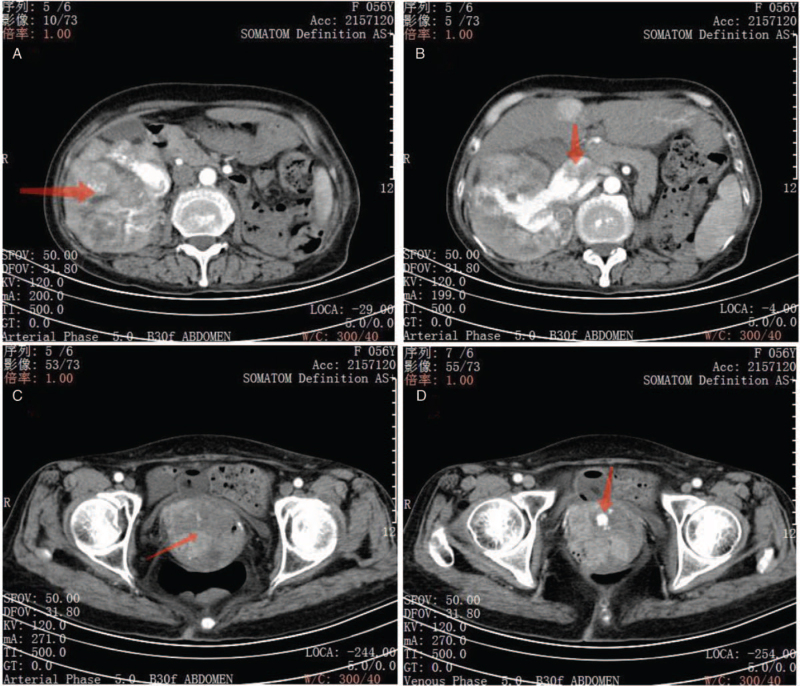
A, Abdominal enhancement CT shows irregular mixed hypodense mass in the right kidney with heterogeneous enhancement. B, Soft tissue mass visible in the vena cava with consideration of cancer embolism. C, Irregular vaginal mass with heterogeneous enhancement. D, Active bleeding within the vaginal mass with pseudoaneurysm formation. CT = computed tomography.
